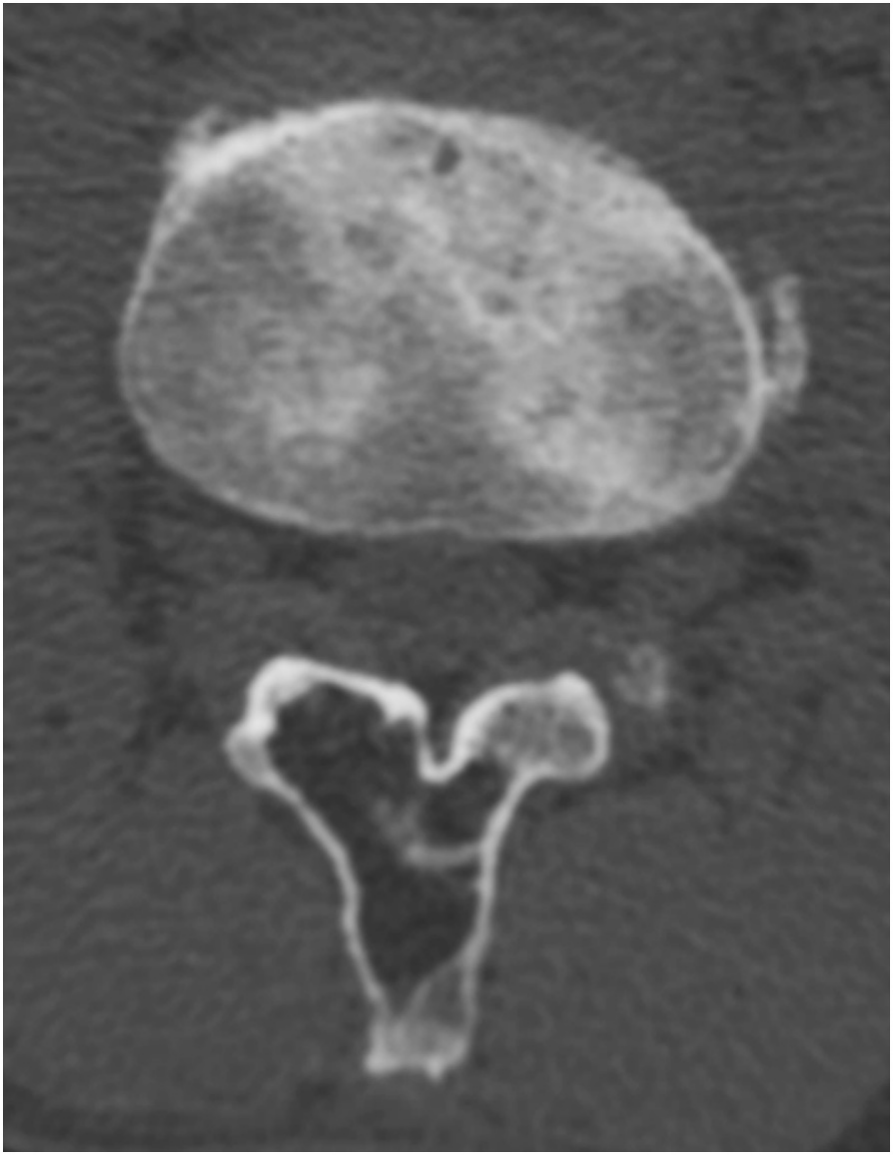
Computed tomography image revealing an osteolytic change accompanied by marginal osteosclerosis in his third lumbar vertebral arch and spinous process, as well as a thinned and bulging bone cortex.
